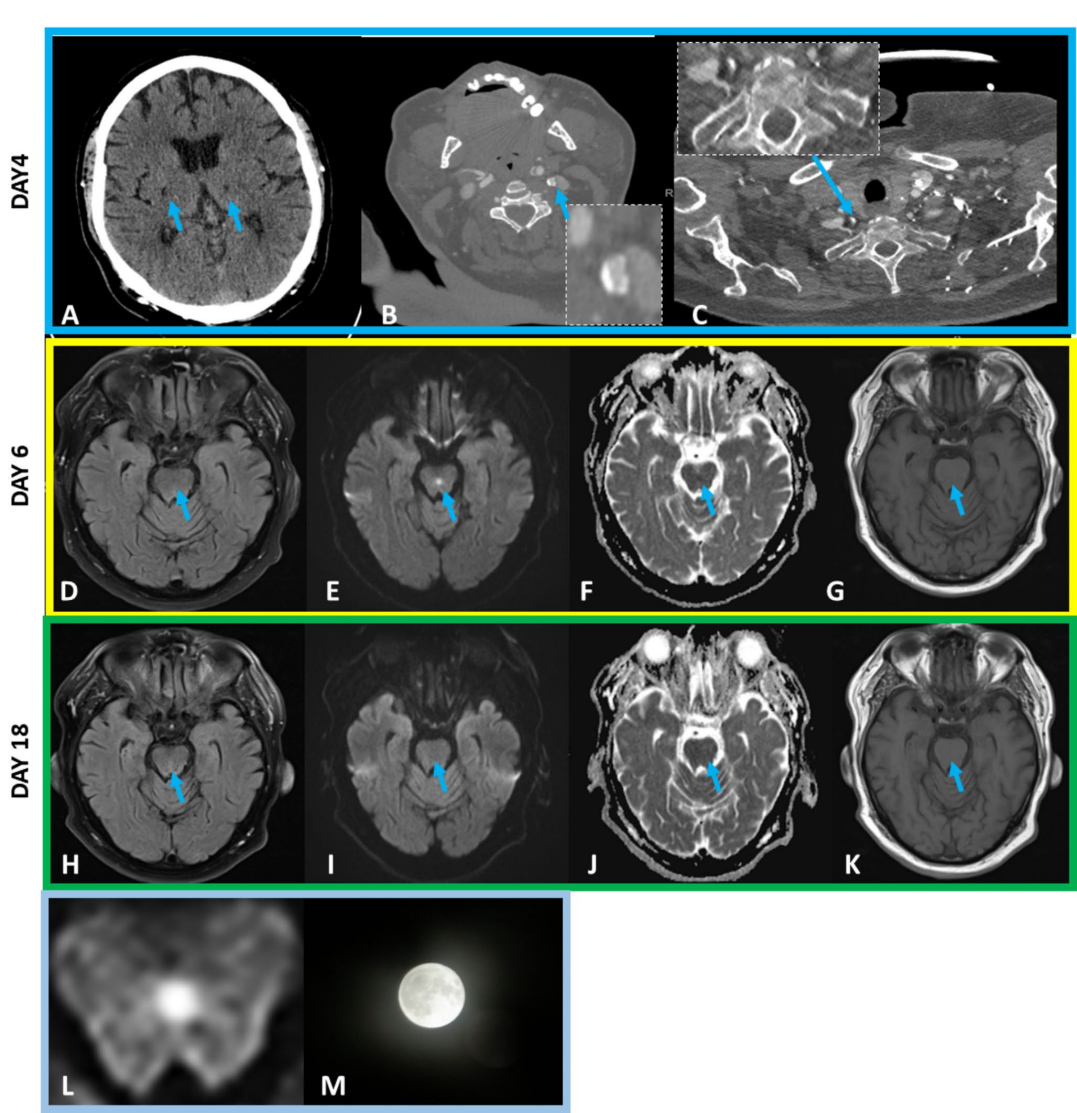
A-L. Neuroradiological features detected in the described patient. A-C Axial Head CT-scan, axial CT-Angiography of Head and Neck, 4 days after onset of symptoms. A, Axial-Head-CT-Scan displaying two old lacunar infarcts (anterior limb of the capsula interna right and in thalamus left, blue arrows). B-C CT-Angiography of Head and Neck showing a moderate stenosis (ca. 50% by NASCET criteria) of the left internal carotid artery (B, blue arrows) and a severe stenosis of the right vertebral artery (V1 Segment) (C, blue arrows). D-G, axial Brain MRI scans performed 6 days after symptom onset and displaying a central, round, symmetric and bilateral lesion close to Wernekink commissure in the mesencephalon with subtle hyperintensity on fluid attenuated inversion recovery (FLAIR) (D) corresponding to a T2-diffusion weighted imaging (DWI) with restricted diffusion (E) and low signal intensity on apparent diffusion coefficient (ADC)(F) and a very mild hypointense signal on T1-weighted imaging (G) (blue arrows).H-K, Follow up brain MRI scans performed 18 days post symptom onset presenting a complete resolution of the midbrain lesion in the T2-FLAIR (H) as well as in the T2- DWI sequences (I), on apparent diffusion coefficient (ADC) (J) and on T1-weighted imaging sequences (K) (blue arrows), corresponding to the patient clinical remission. L-M. T2- Diffusion*-*weighted imaging (DWI) performed 6 days after onset of symptomatic, presenting a midbrain central, symmetric, round lesion with restricted diffusion (L), typically resembling the shape of a full moon (M)
